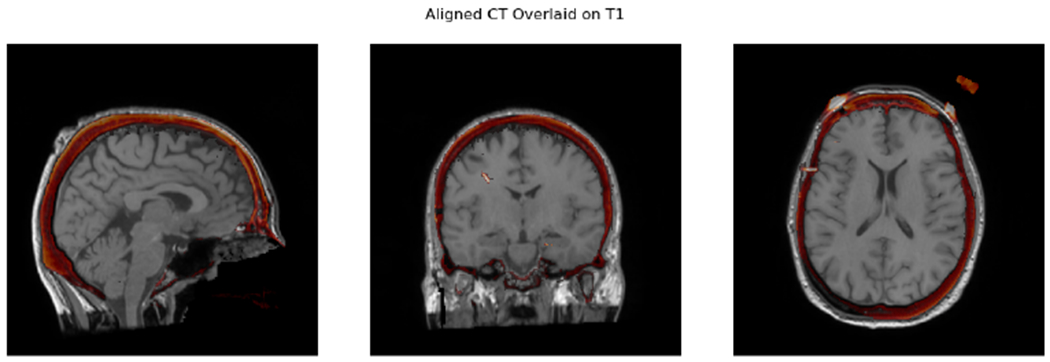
A CT image correctly aligned to the MR image. The darker areas in the MR are the skull whereas the lighter, outermost regions are subcutaneous fat. The CT is shown with a red scale, and red areas shown in the slice are the skull, which has high intensity in the CT.
